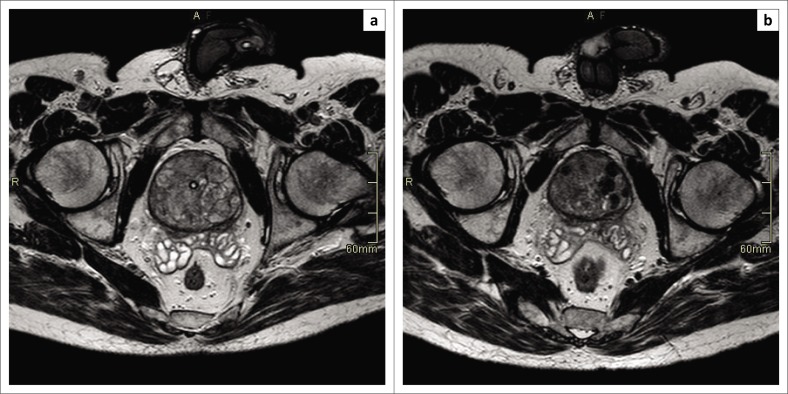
Magnetic resonance imaging of the prostate before and after prostate artery embolisation: (a) Magnetic resonance imaging before prostate artery embolisation with a transurethral catheter in situ. (b) Magnetic resonance imaging 3 months after prostate artery embolisation with the transurethral catheter removed and low-signal intensities in both prostate lobes because of ischaemia.
